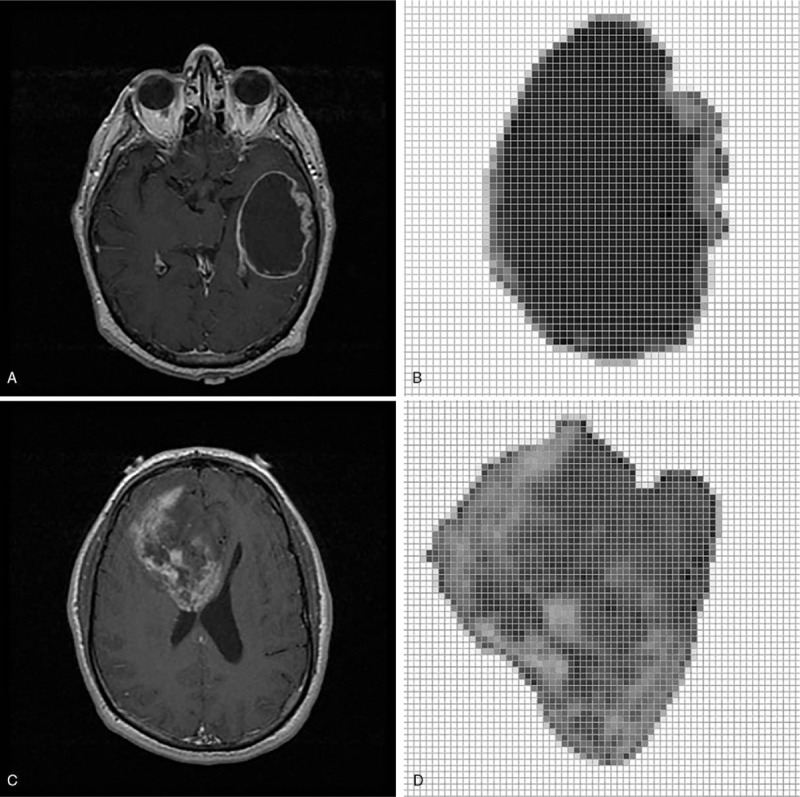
A wild-type (WT) isocitrate dehydrogenase (*IDH*) (A) and a mutant *IDH* genes (C) in magnetic resonance image. (B) and (D) are extracted tumors (http://cancerimagingarchive.net/; “License” and the CC BY license, https://creativecommons.org/licenses/by/3.0/).
